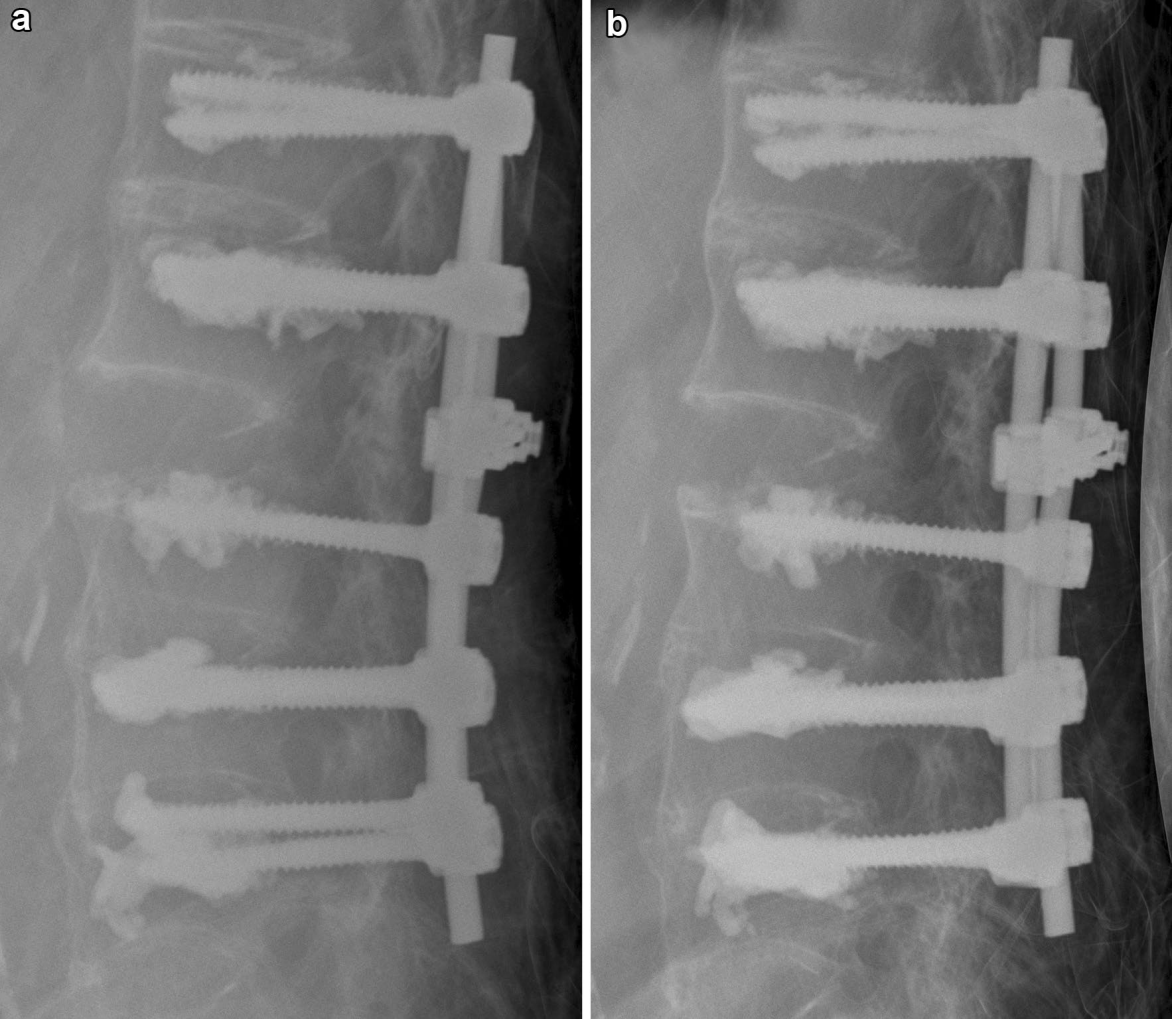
Postoperative X-ray control of the same patient prior to mobilisation (a) and after 3 weeks (b). No restoration of pre-injury sagittal alignment
